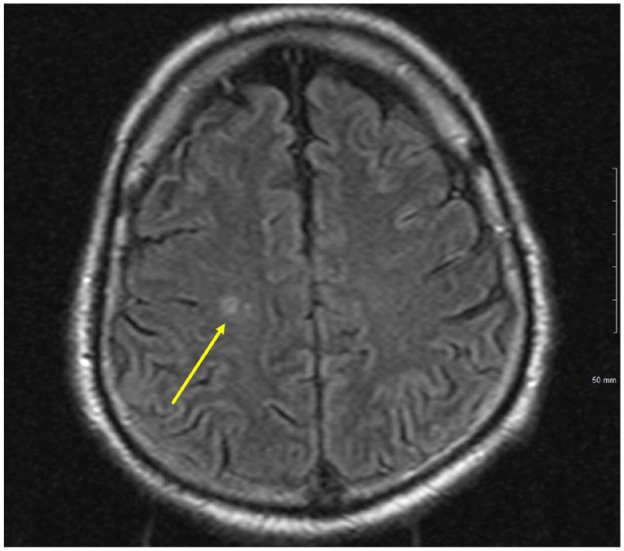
CT chest: right cavitary lesion 2.4 cm × 1.7 cm.
